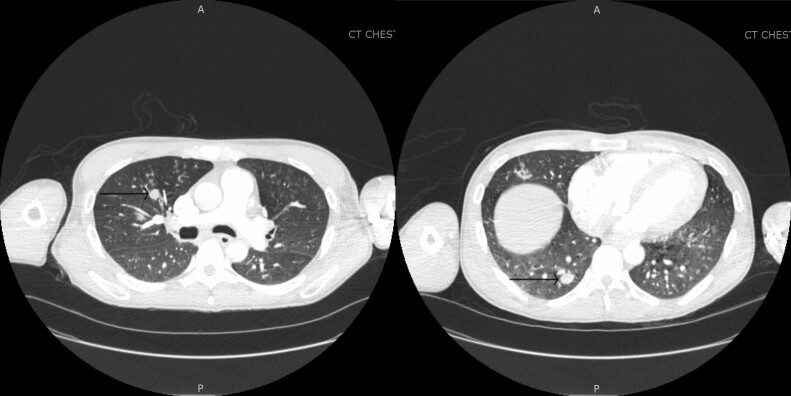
Patient's chest computed tomography (CT) at presentation. Arrows are showing multiple lung nodules of varying sizes.
